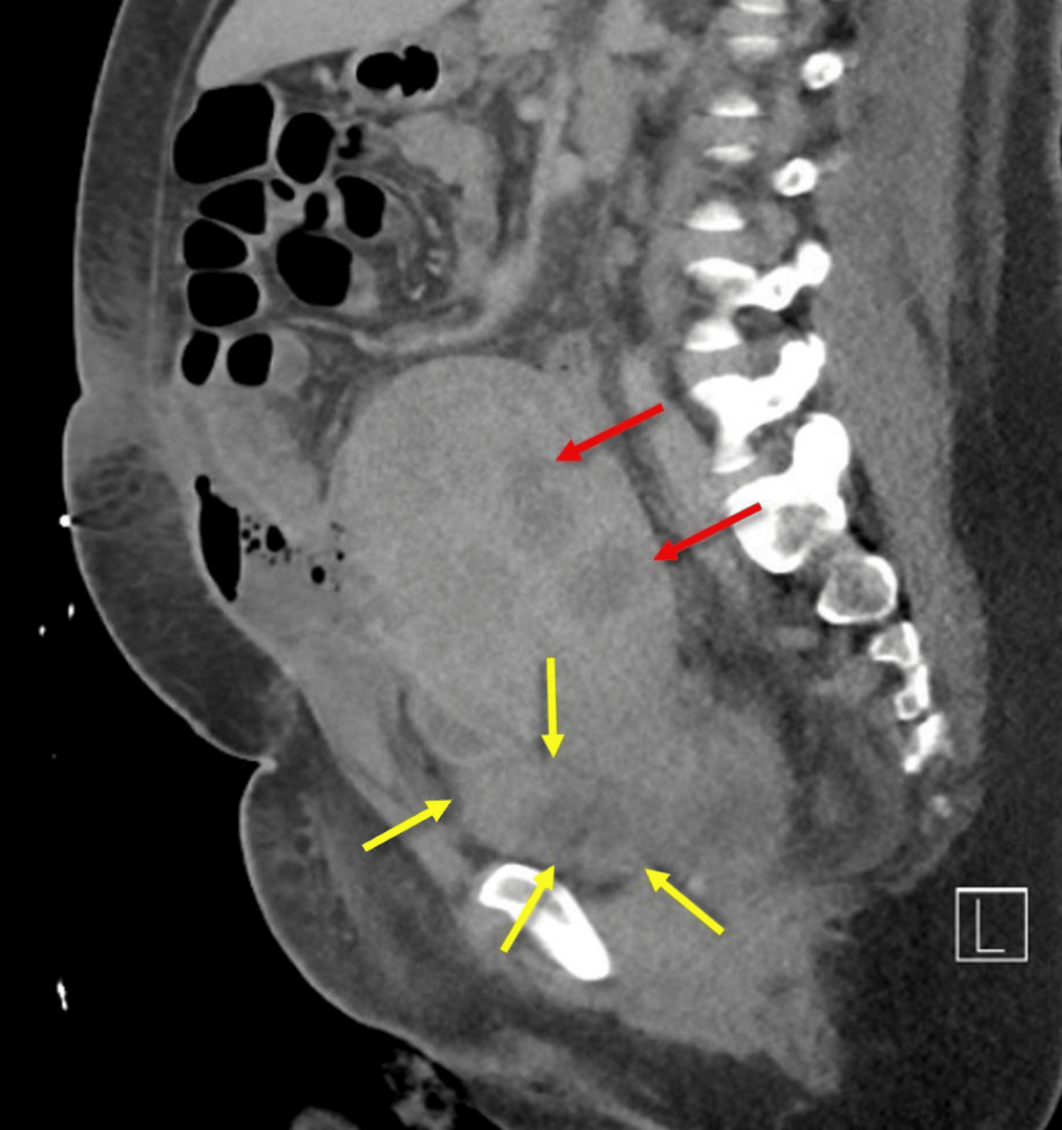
CT of the abdomen and pelvis (sagittal plane) The yellow arrows show fluid collection that is contiguous with the anterior uterine wall in the region of the surgical site. Intraperitoneal abscess, postoperative seroma, and resolving hematoma were all in the differential diagnosis. The red arrows show the nodularity in the uterine wall consistent with myomas. Intrauterine abscess and endometritis could not be excluded.
